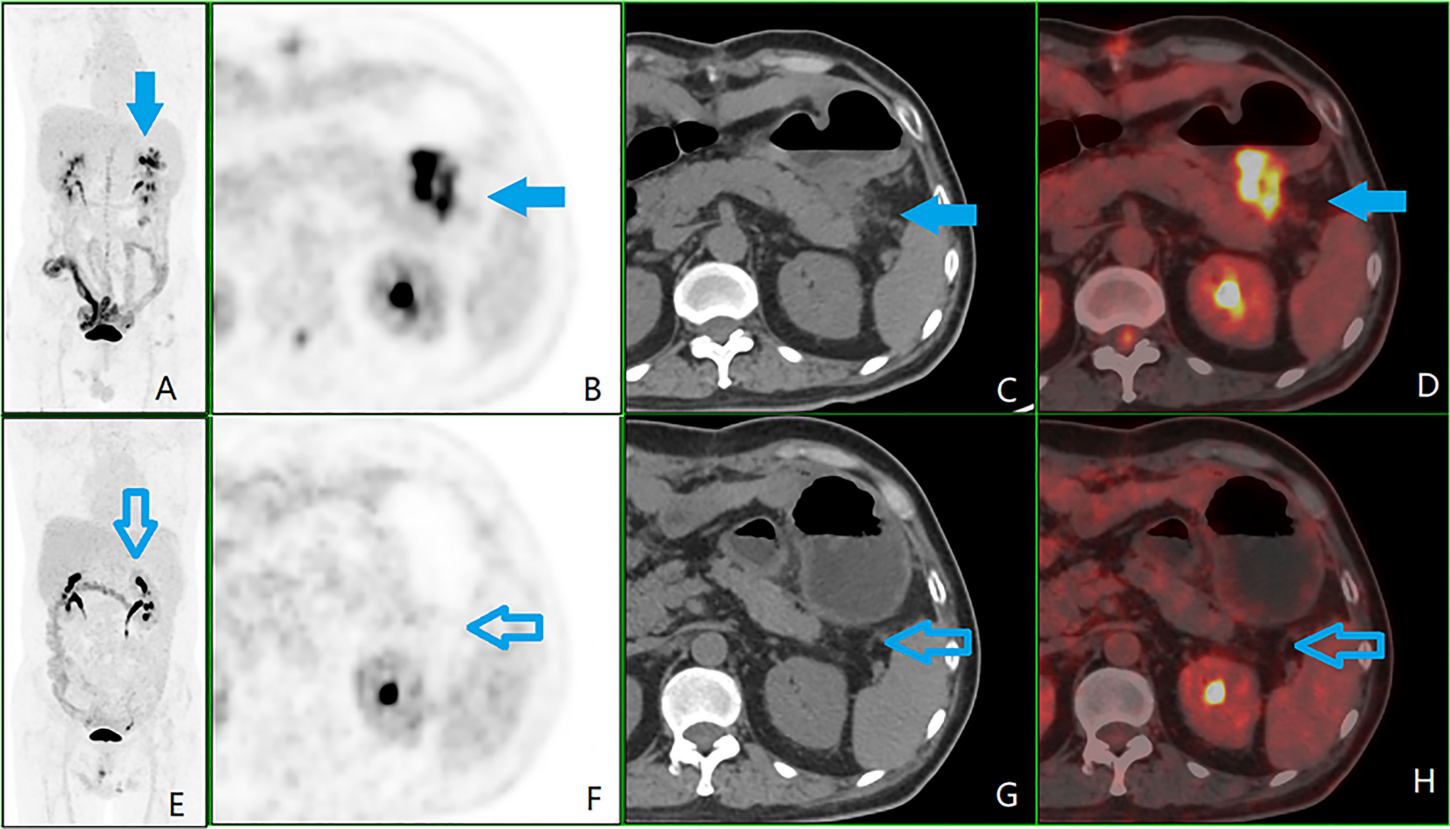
A patient with colorectal peritoneal carcinomatosis (CRPC), male, 56 years old, operated on for ulcerative poorly differentiated adenocarcinoma of the descending colon on May 17, 2017. (A) Positron emission tomography (PET) 3D-MIP image; (B) PET cross-sectional image; (C) computed tomography (CT) cross-sectional image; (D) PET/CT fusion cross-sectional image; (E–H) and (A–D) are in the same order, which is of the second ^18^F-fluorodeoxyglucose PET/CT examination on September 11, 2018, after six courses of chemotherapy. The first examination showed plaques with soft tissue density, i.e., CRPC lesions (shown by the blue arrow) in the left upper abdominal region (zone 3) next to the anterior tail of the pancreas, lesion size 2, with a peritoneal cancer index score of 2. The glucose metabolism was significantly elevated, with a standardized uptake maximum value of 20.5, a time-lapse period of 31.8, and a retention index of 55.1%. A review after completion of six courses of chemotherapy showed a significantly reduced, undefined CRPC lesion (shown by the hollow blue arrow).
